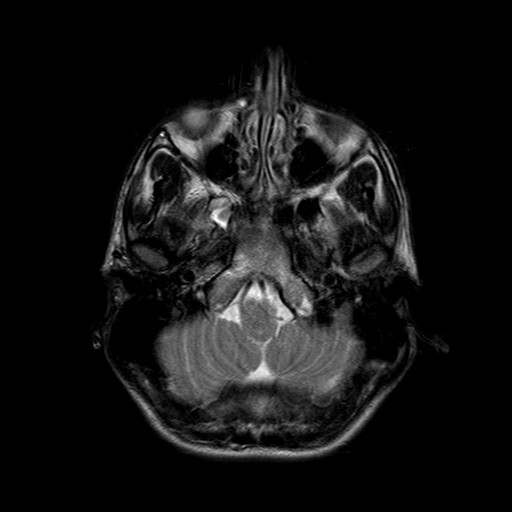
Transverse T2-weighted MRI brain image shows small high-signal lesions on the lateral right side of the pontine tegmentum.
